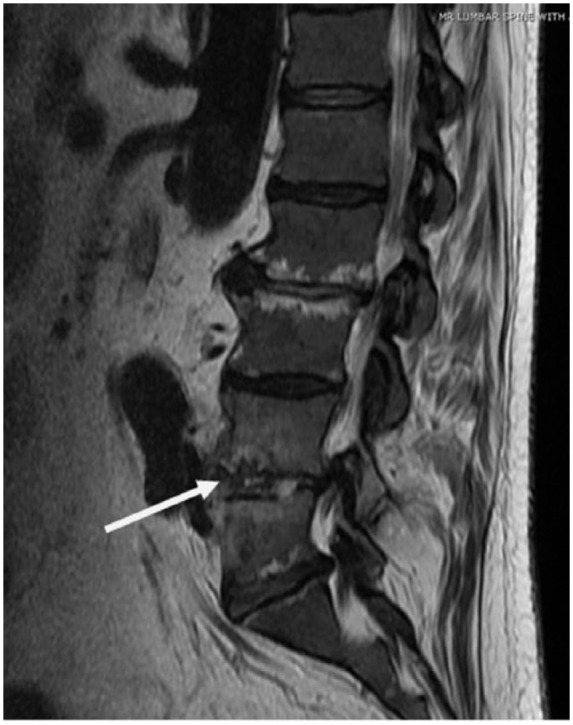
Magnetic resonance imaging: Discitis/osteomyelitis at L4-L5 with preservation of vertebral body height but an extension of infection into the epidural space, as well as anteriorly and into the left posterior paraspinal soft tissues.
